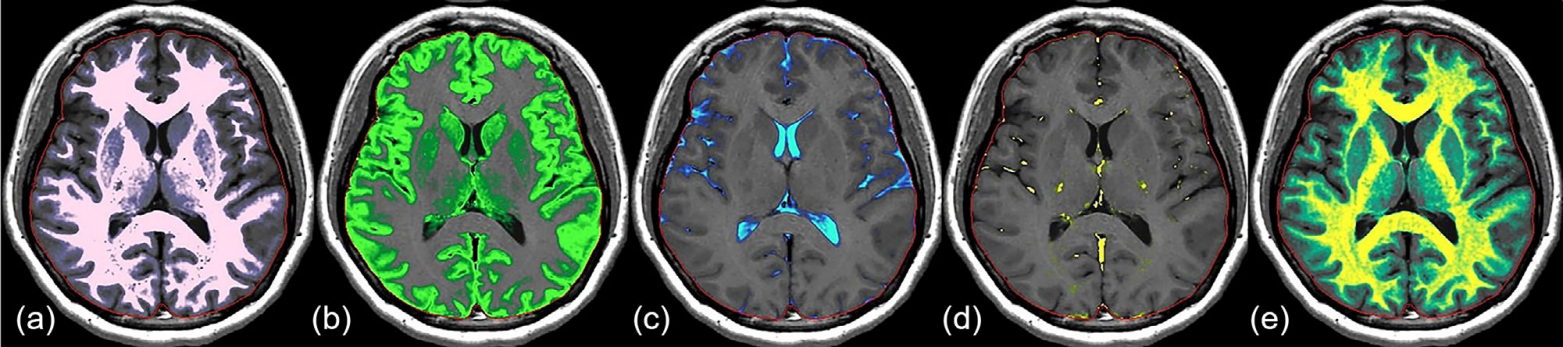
An example of brain tissue segmentation using synthetic magnetic resonance imaging (MRI). white matter (WM), (a), gray matter (GM) (b), cerebrospinal fluid volume (CSF) (c), non‐WM/GM/CSF (NoN) (d), and myelin (MY) (e) maps are overlaid on a synthetic T1‐weighted image (TR/TE = 500/10 msec).
